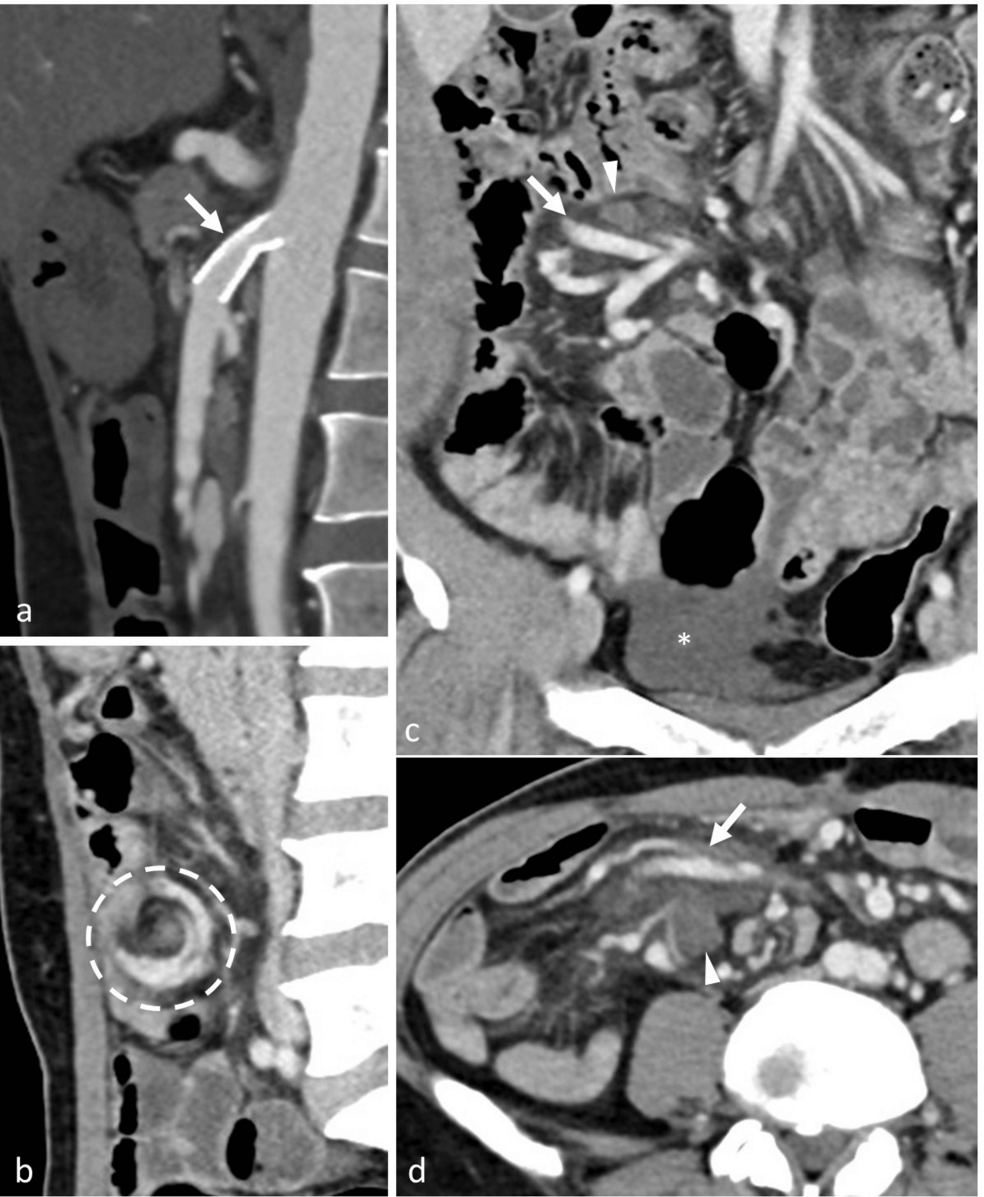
Venous congestion in the hernia sac after SMA stenting (a) Sagittal reformatted arterial phase CT image showing patent SMA stent (arrow). (b) Sagittal reformatted venous phase CT image showing persistent volvulus of the hernia sac (outlined by dashed circle). (c, d) Coronal reformatted (c) and axial (d) venous phase CT images showing engorgement of the mesenteric veins (arrows), lymphadenopathy (arrowheads), and fat stranding in the hernia sac along with mild ascites in the pelvis (asterisk in c) SMA: superior mesenteric artery; CT: computed tomography
